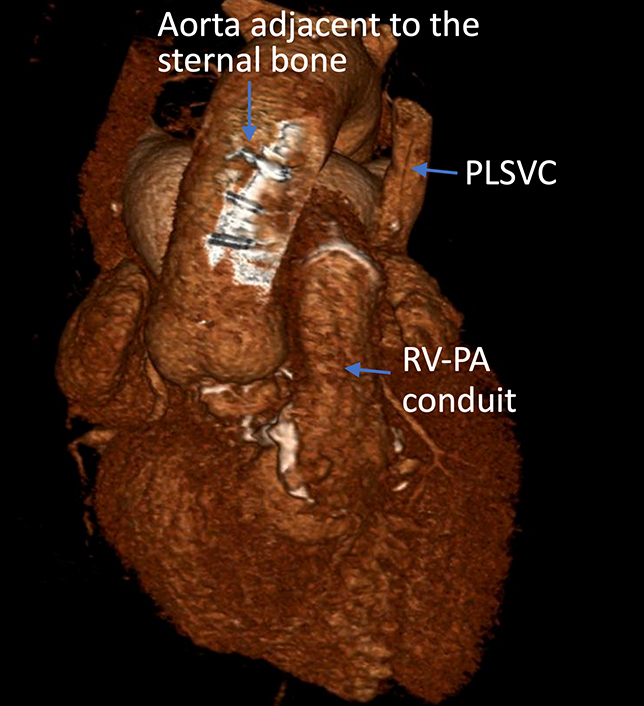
Three-dimensional reconstruction of computed tomography scan.
